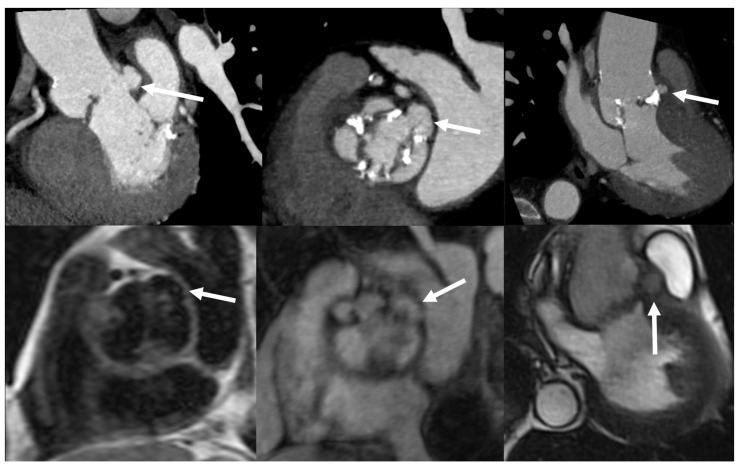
Paravalvular abscesses. CCTA (top images) multiplanar reconstructions and Cardiovascular Magnetic Resonance (CMR) images (bottom images: T1-weighted Turbo Spin Echo on the left, contrast-enhanced T1-weighted Gradient Echo 3D image in the middle and cine-CMR on the right) show a diffuse, partially calcified, thickening of the aortic valve leaflets in a 58-year-old man with *Staphilococcus aureus* IE and bicuspid valve. IE was complicated by the formation of small perivalvular abscesses, which, following the opening of their contents in the lumen, appear as little saccular outpouchings (arrows).
